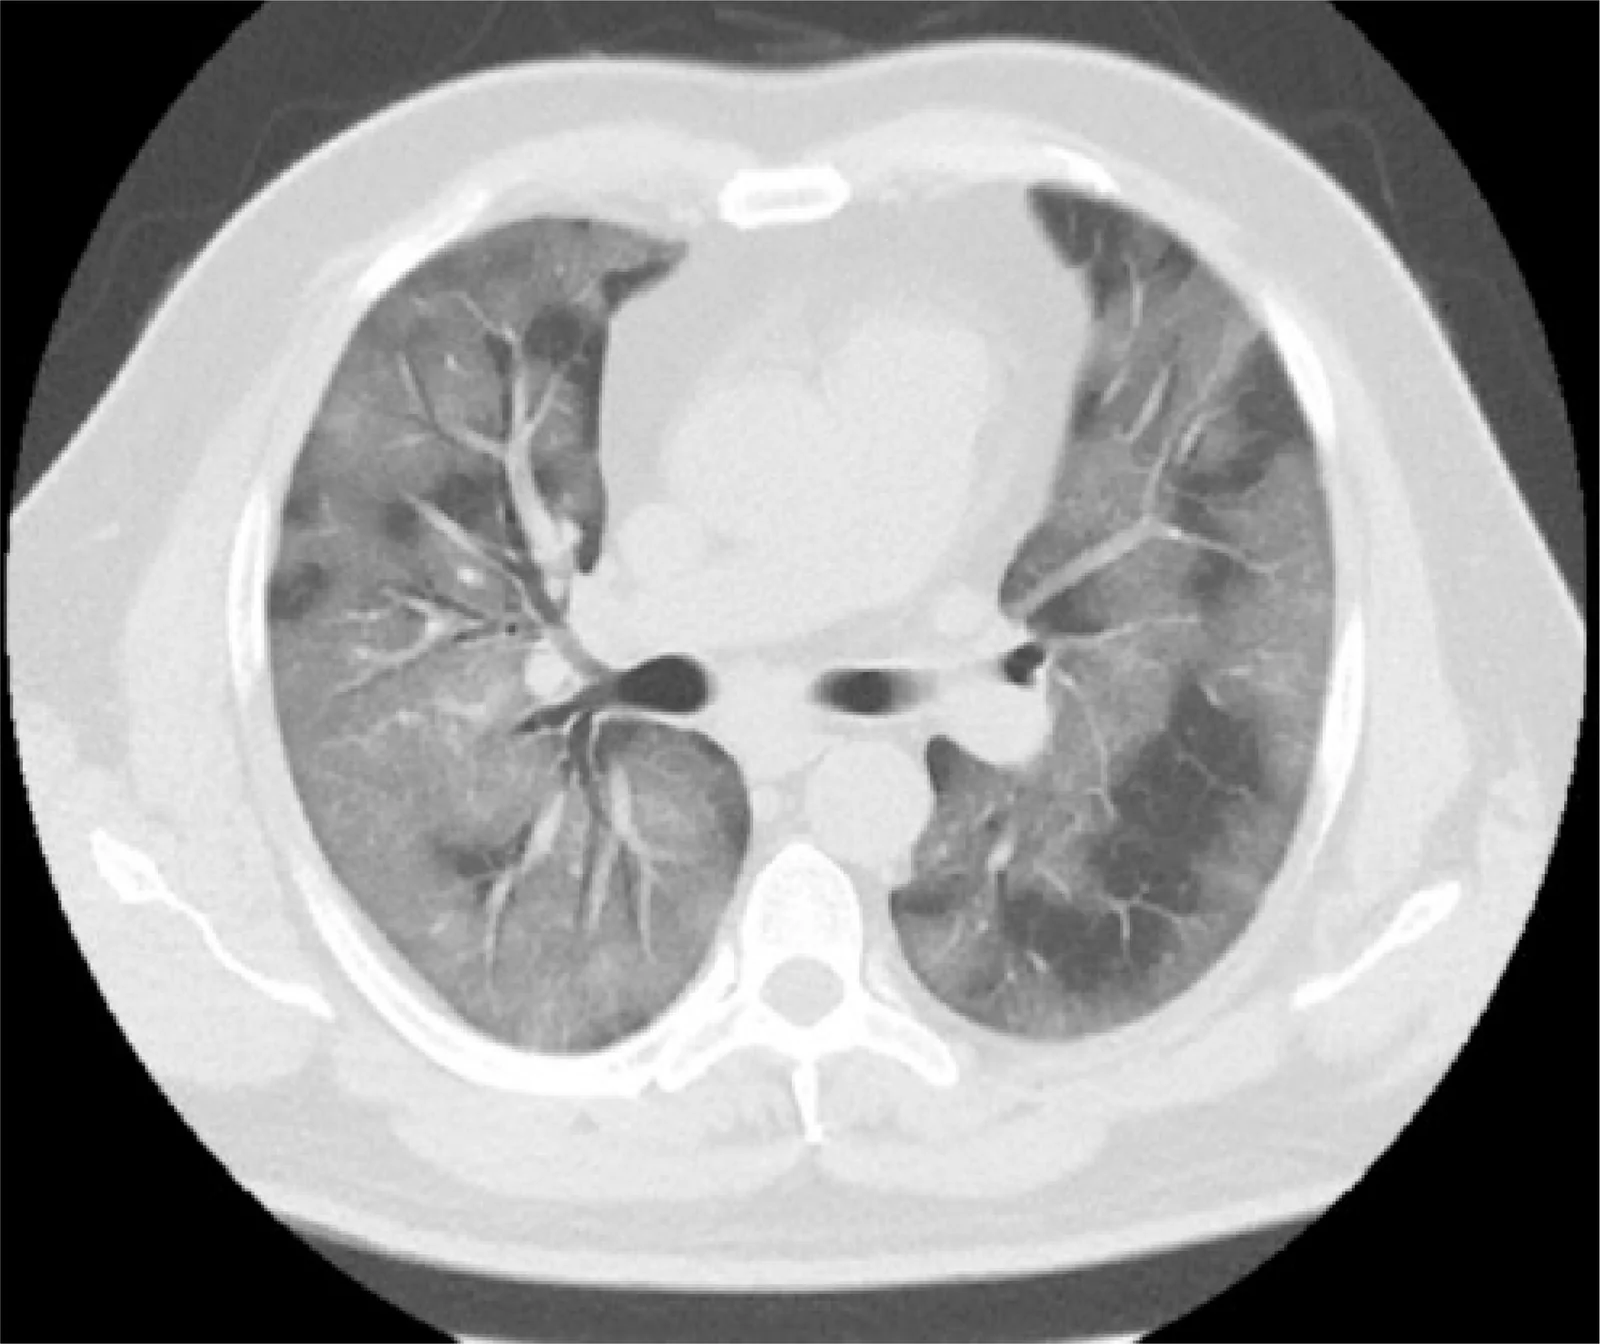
A 60-year-old male patient with COVID-19 pneumonia. Multifocal ground-glass opacities are seen in the bilateral lung parenchyma. Lung involvement is about 90% (CT severity score 5). (Courtesy: Government Base Hospital, Almora, Uttarakhand).
